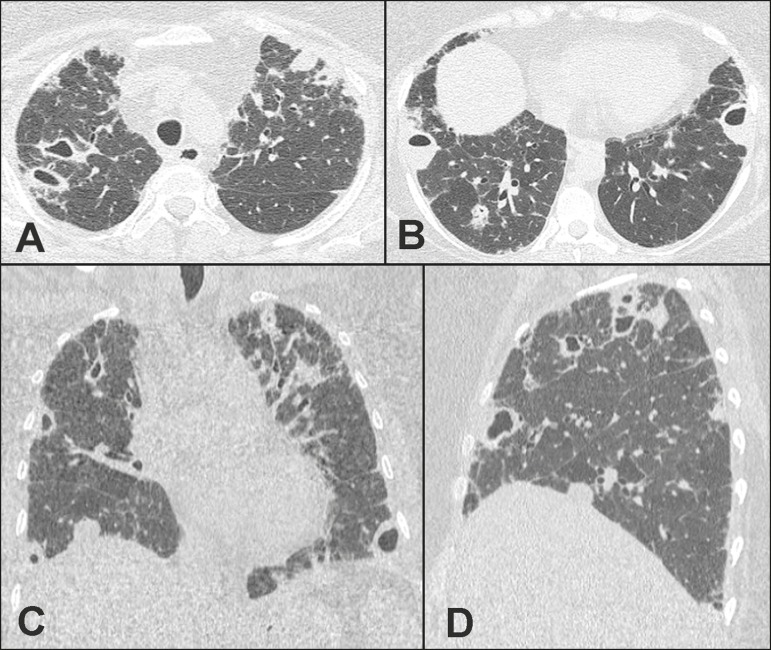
Computed tomography of the chest, in axial views (A,B), a
